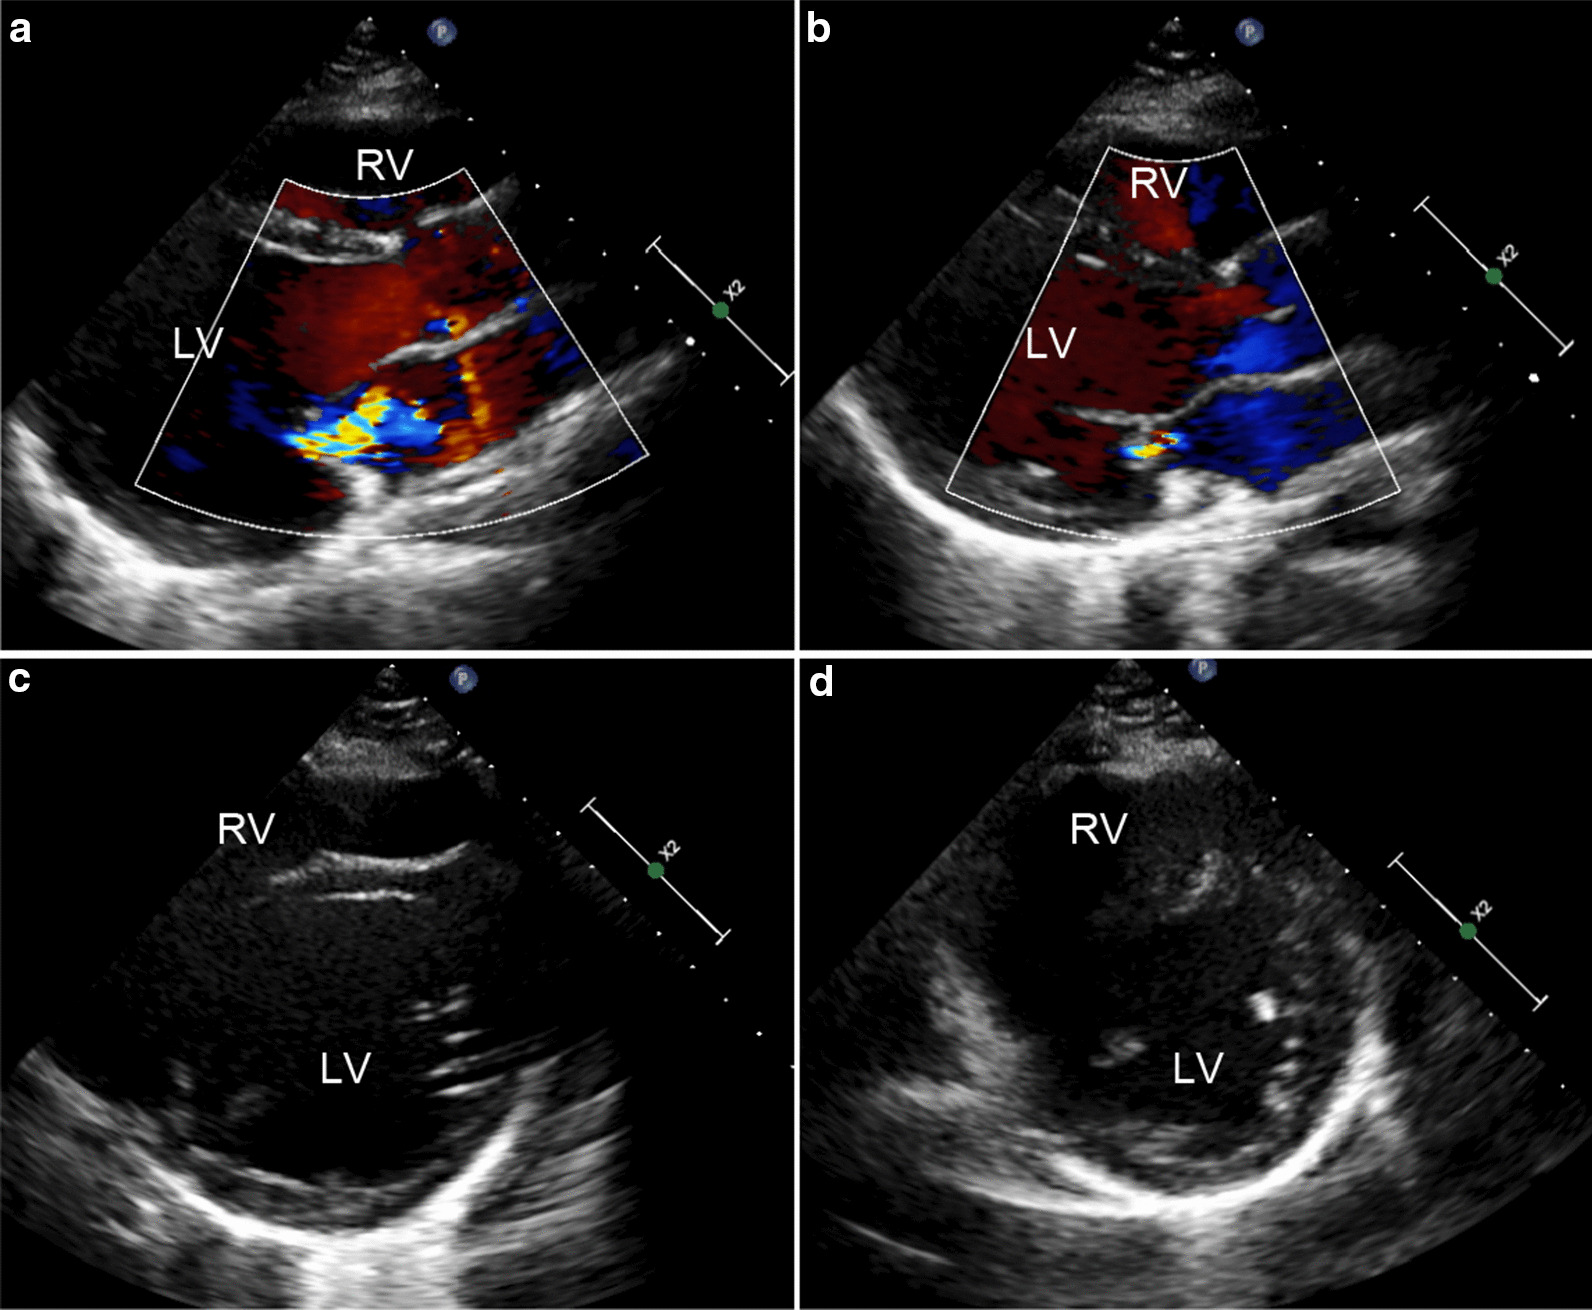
Effects of amiodarone treatment on echocardiographic parameters. Color Doppler TTE in parasternal long-axis view (a, b), and TTE in parasternal short-axis view (c, d). Echocardiography (day 4) demonstrates severe dilated cardiomyopathy with moderate MR (LVEF, 27%; LVDD, 61 mm) (a, c). Follow-up echocardiography (day 38) demonstrates significant improvement in ventricular function and MR (LVEF, 61%; LVDD, 37 mm) (b, d). LV: Left ventricle, LVEF: Left ventricular ejection fraction, LVDD: Left ventricular diastolic diameter, MR: Mitral regurgitation, RV: Right ventricle, TTE: Transthoracic echocardiography
